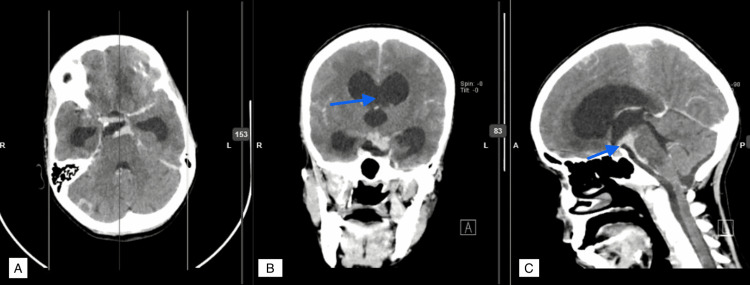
Preoperative CT brain contrast study (A - axial, B - coronal, C - sagittal sections) of the same patient showing dilated bilateral lateral and third ventricle with minimal periventricular seepage and leptomeningeal enhancement.
